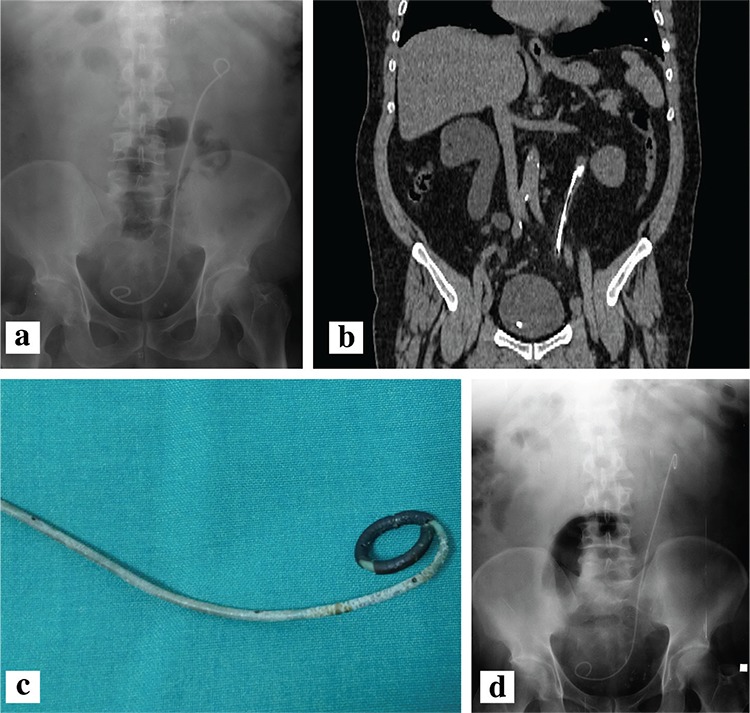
Some images of a patient whose double-J stent had remained in the body for 30 months. Thickened stent due to encrustation (a). Encrustations and stones on computerised tomography, which were not seen in the KUB radiograph (b). Encrustation on the stent; stent was removed by ureteroscopy (c). A new stent inserted in the same patient at the same calibration (6 Fr.) (d). Notice the difference between this new stent and the thickened encrusted stent.
